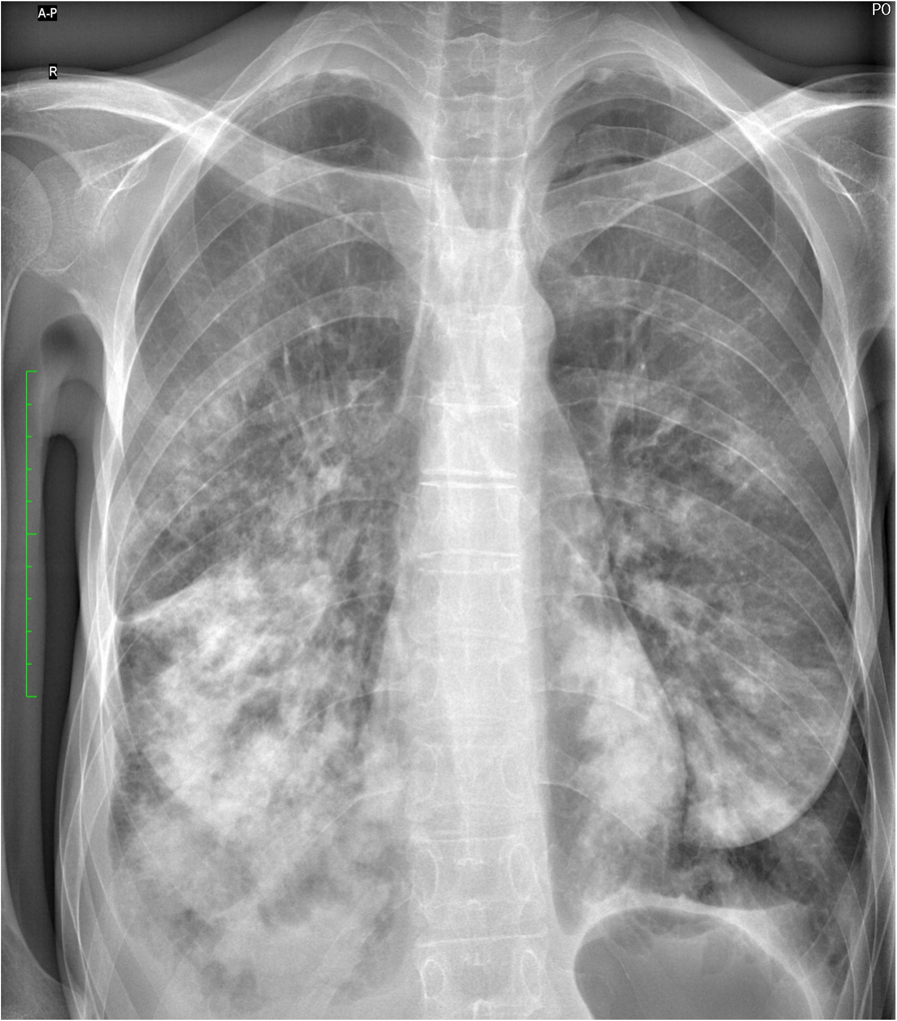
Subsequent chest X-ray revealing a similar state of the right lung compared to the previous examination
